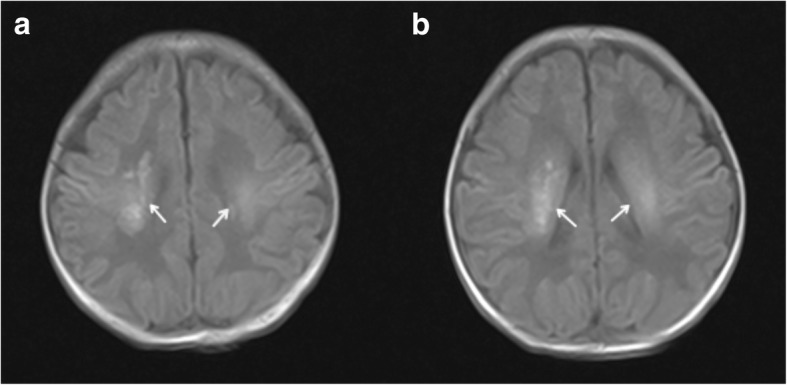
Head MRI of T1 WI axial images of life 5. a and b demonstrate asymmetric hyperintense changes in centrum semiovale and periventricular white matter, especially on the right side (see arrows)
